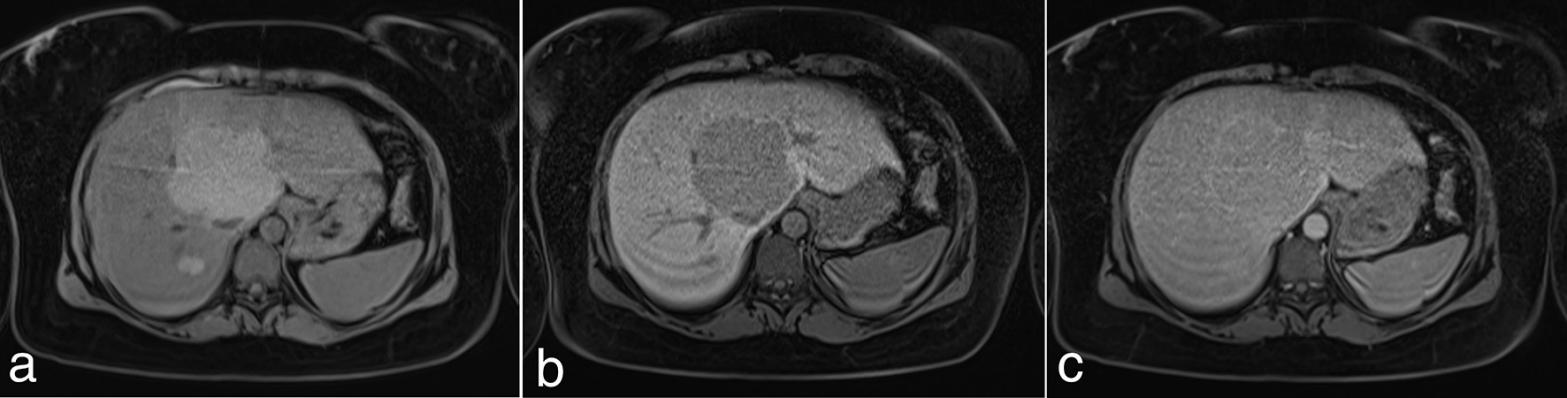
Dynamic MRI August 2012. (a) Pre-Primovist (b) Hepatobiliary phase (c) Equilibrium phase displaying pseudocapsule appearance.
